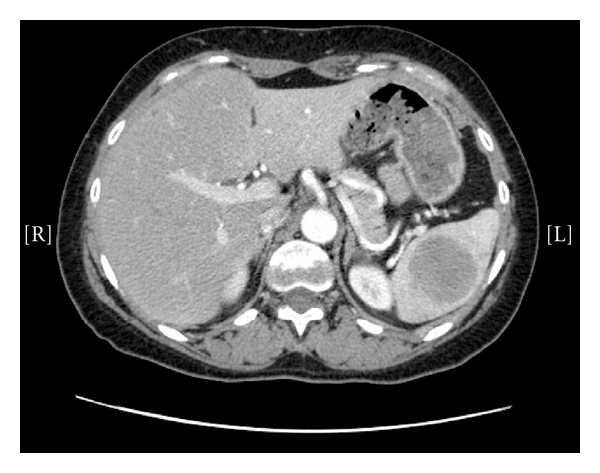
CT scan showing a 4.7 cm solitary splenic lesion.
